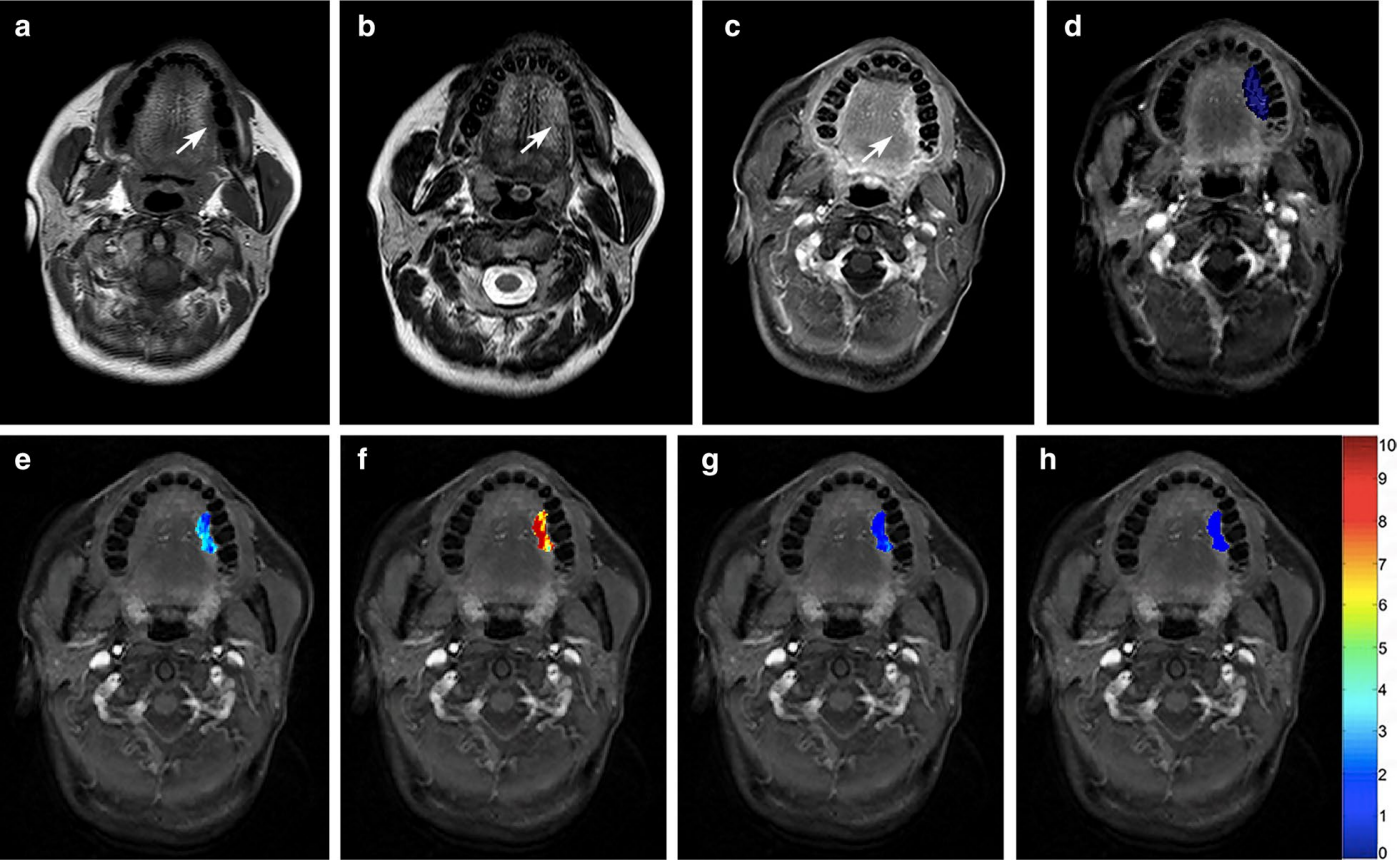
A well differentiated squamous cell carcinoma in the tongue, T1N0M0 (stage I). a–c The tumor was seen as a 20 mm × 12 mm × 11 mm mass (arrows) with isointense signal on T1WI (a) and hyperintense signal on T2WI (b), with heterogeneous enhancement on fat-suppressed contrast-enhanced T1WI (c). d DCE-MRI parameters are evaluated by a 3-D volume of interest (arrow). e–h Pseudocolorized maps show individual parameters derived from DCE-MRI. The measured K^trans^ (e) is 0.125 min^−1^, K_ep_ (f) is 0.995 min^−1^, V_e_ (g) is 0.127, and V_p_ (h) is 0.054
